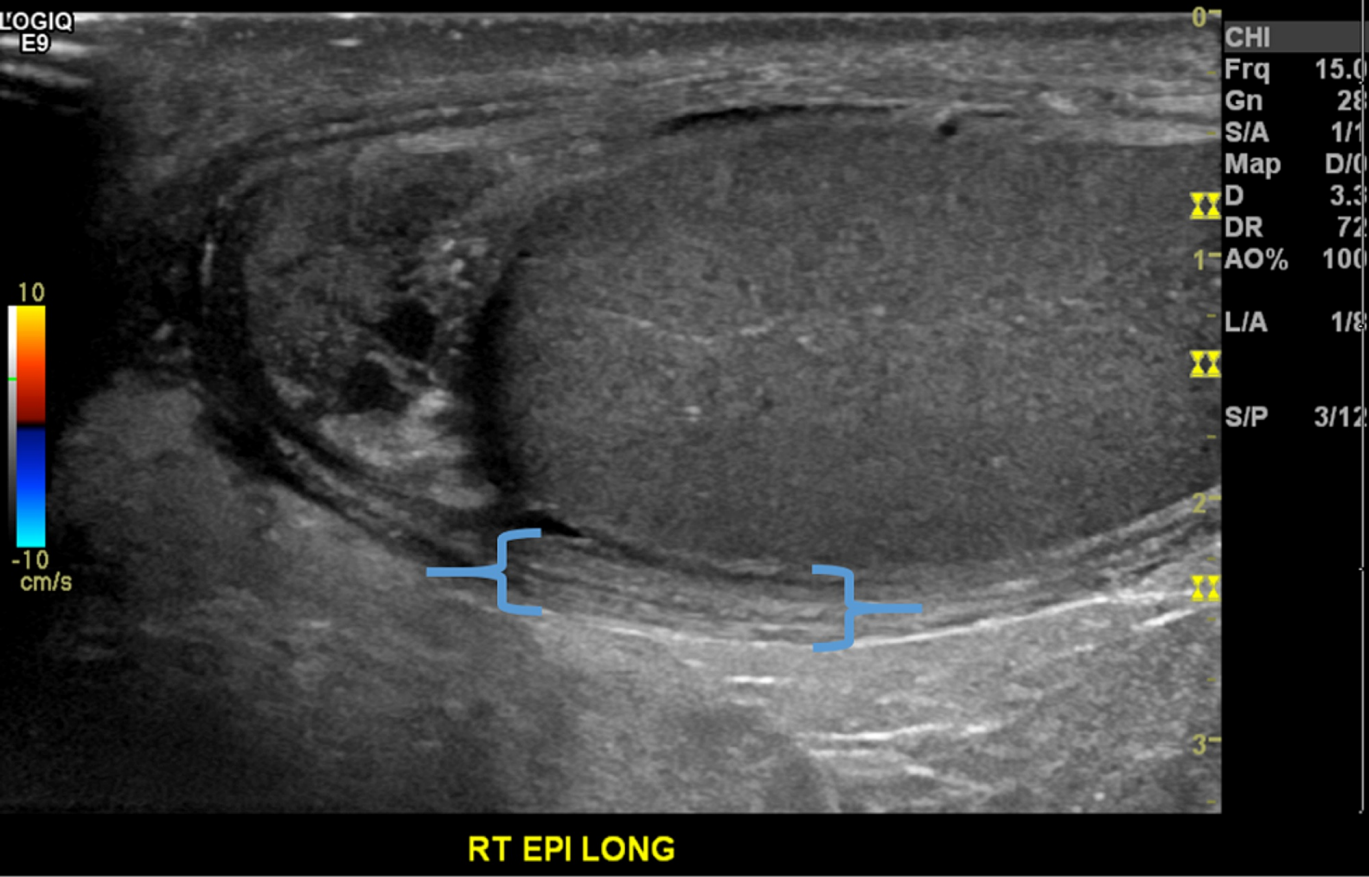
Long grey-scale ultrasonography of the right hemi-scrotum Blue brackets: significant scrotal edema
